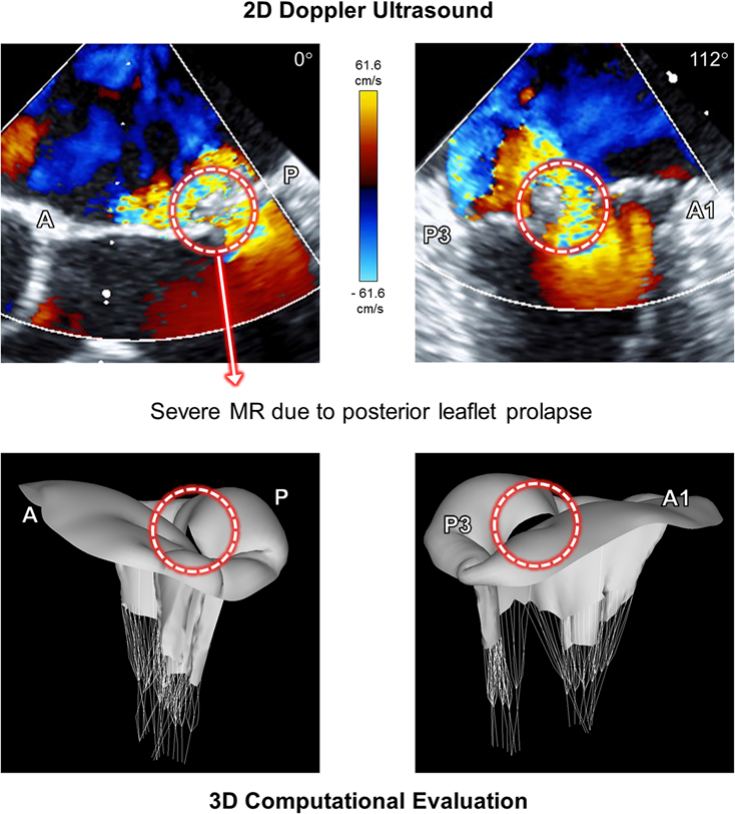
Doppler ultrasound images and the corresponding 3D morphologies of the MV of the pathologic MV involving posterior chordal rupture. Data shown at peak systole.
